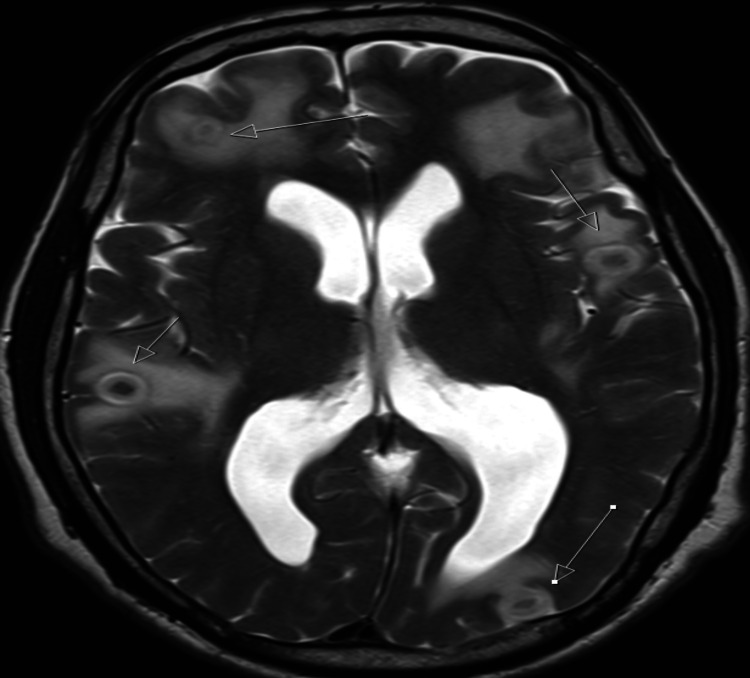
MRI showing multiple tuberculomas
